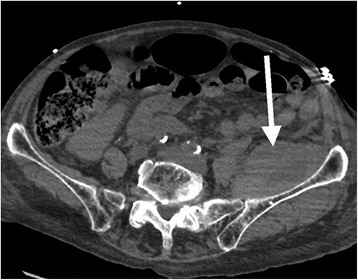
Abdominal CT scan. Hematoma of the left iliopsoas (White arrow).
